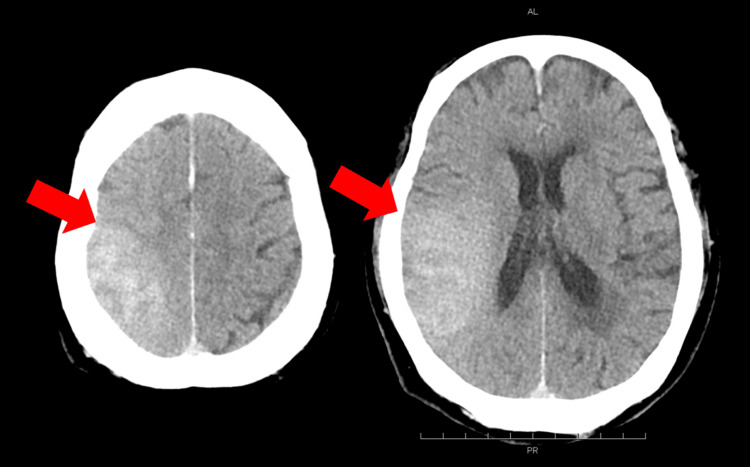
Head CT scan performed immediately after dialysis A CT scan performed immediately after dialysis confirmed the disappearance of the high-density areas (arrow).
